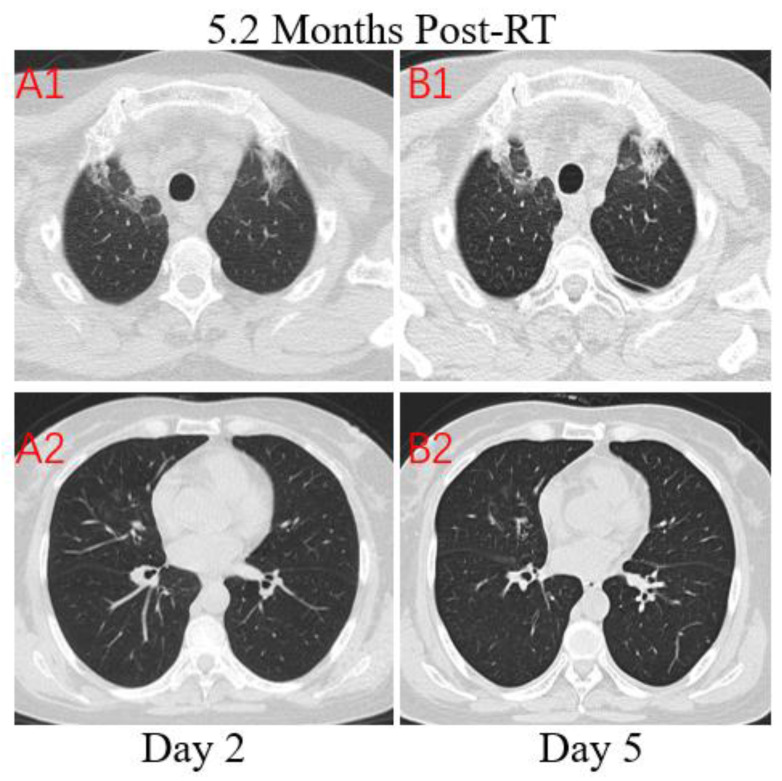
Transverse unenhanced thin-section serial CT scans from a 64-year-old female with suspected COVID-19 pneumonia. Chest CT images on the second day after symptom onset found minimal ground-glass opacities with partially rounded consolidation in the apexes of both lungs (A1), and multiple ill-defined patchy ground-glass opacities in the middle lobe of right lung (A2). Follow-up CT on the fifth day demonstrated no obvious change of lung lesions (B1, B2).
